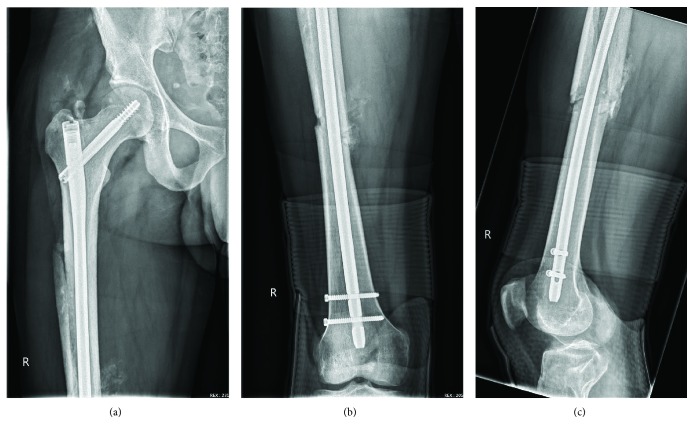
Postoperative anteroposterior radiograph of the right femur (a, b); postoperative lateral radiograph of the right knee (c).
